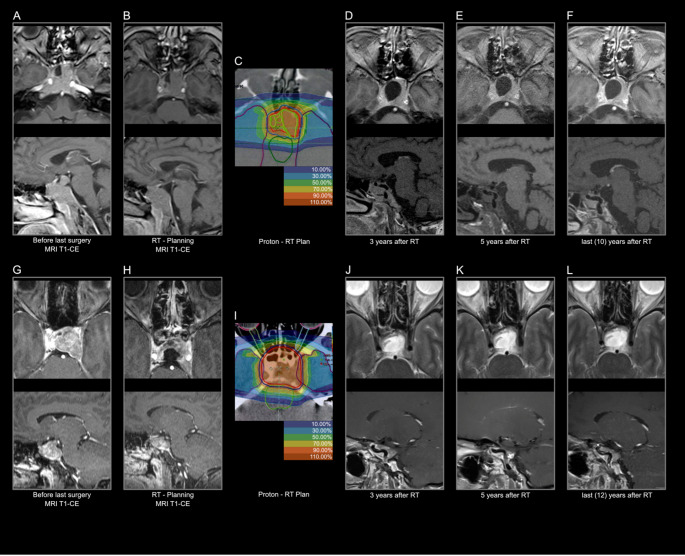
Tumor control of pituitary adenoma (PA) following adjuvant proton radiotherapy (PRT). Representative cases of two patients (PA-04: A–F; PA-05: G–L) with longest available MRI follow ups of 10 and 12 years, respectively, shown using MRI in axial and sagittal sections. (A, G): T1-weighted contrast-enhanced MRI before the last surgery. (B, H): MRI used for planning of additive PRT, depicting residual tumors as contrast-enhancing sellar lesions. (C, I): PRT treatment plan with isodose lines (% of total dose by color wash). Proton delivery via two opposing fields. Follow-up MRI (D, J): 3 years (E, K): 5 years (F, L): and last available post-PRT demonstrating durable tumor control and reduced tumor size in the sellar region
